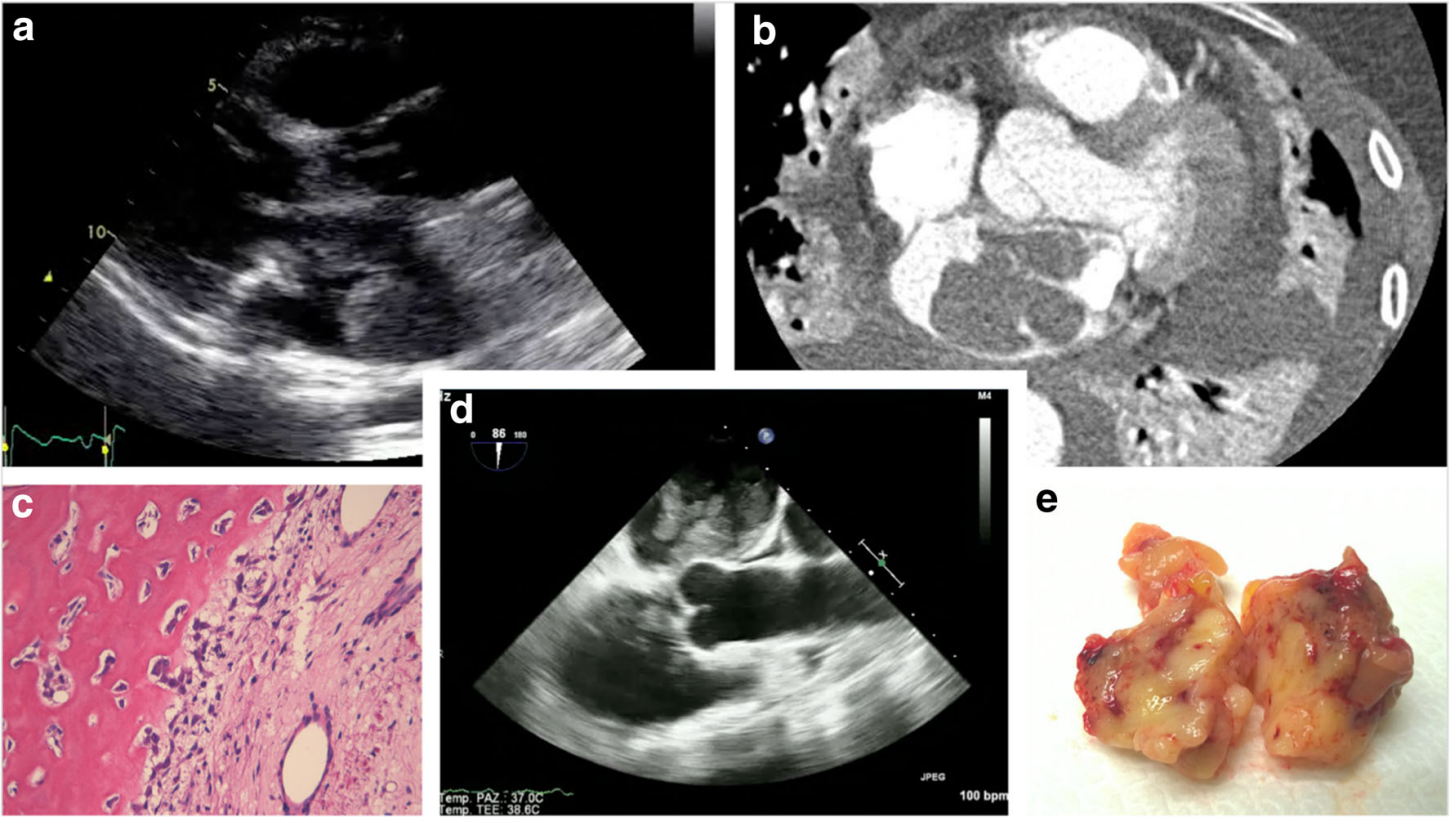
Giant liposarcoma in the left atrium. a TTE long axis section shown left atrium mass with homogenous echogenicity. b CT scan. c Dedifferentiated liposarcoma with osteogenic areas (EE × 20). d TEE long axis view. e Liposarcoma after surgical abscission
